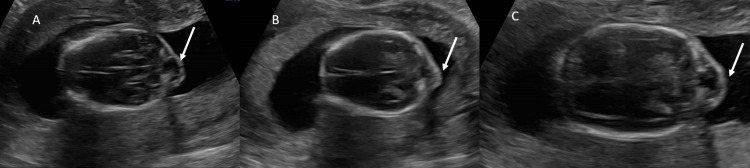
Prenatal Ultrasound: Chiari III Malformation Ultrasound axial image of the brain (A-C) performed at 17 weeks six days of gestation shows occipital cephalocele (arrows)
